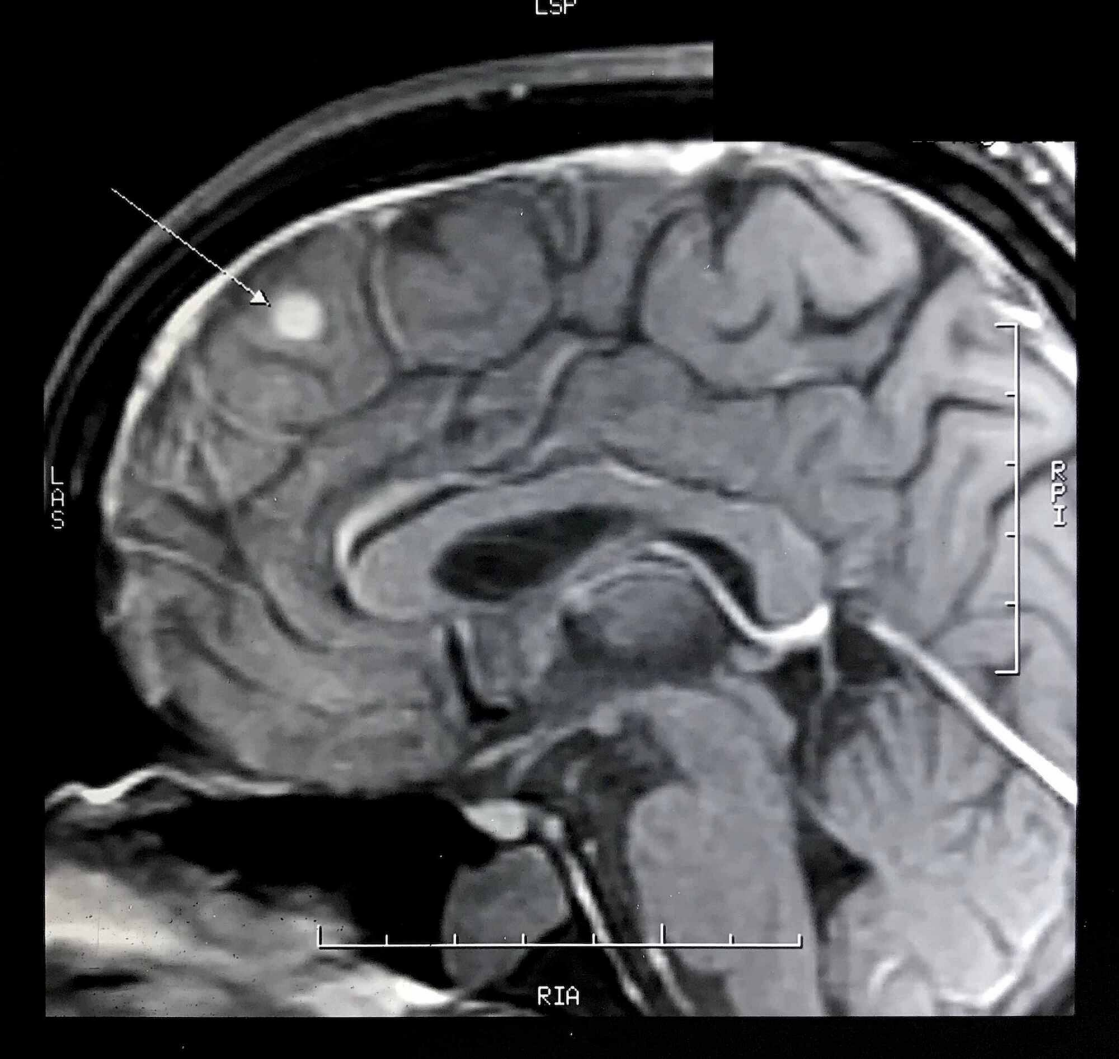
Neurocysticercosis in the right frontal lobe of the brain on MRI.
